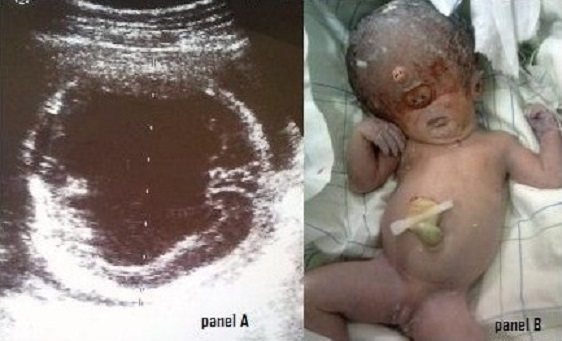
A) Ultrasound scan of the fetal head; B) Postnatal picture of the malformed infant
